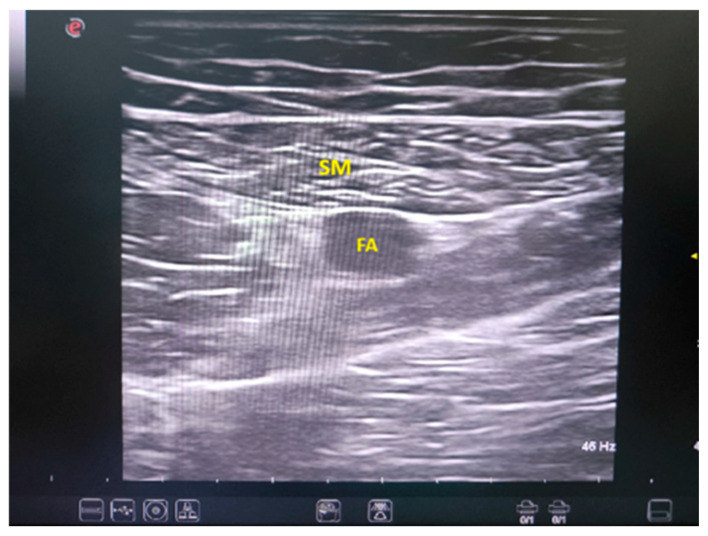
Ultrasound Image of the Mid Adductor Canal Block (FA: Femoral artery, SM: Sartorius muscle).
